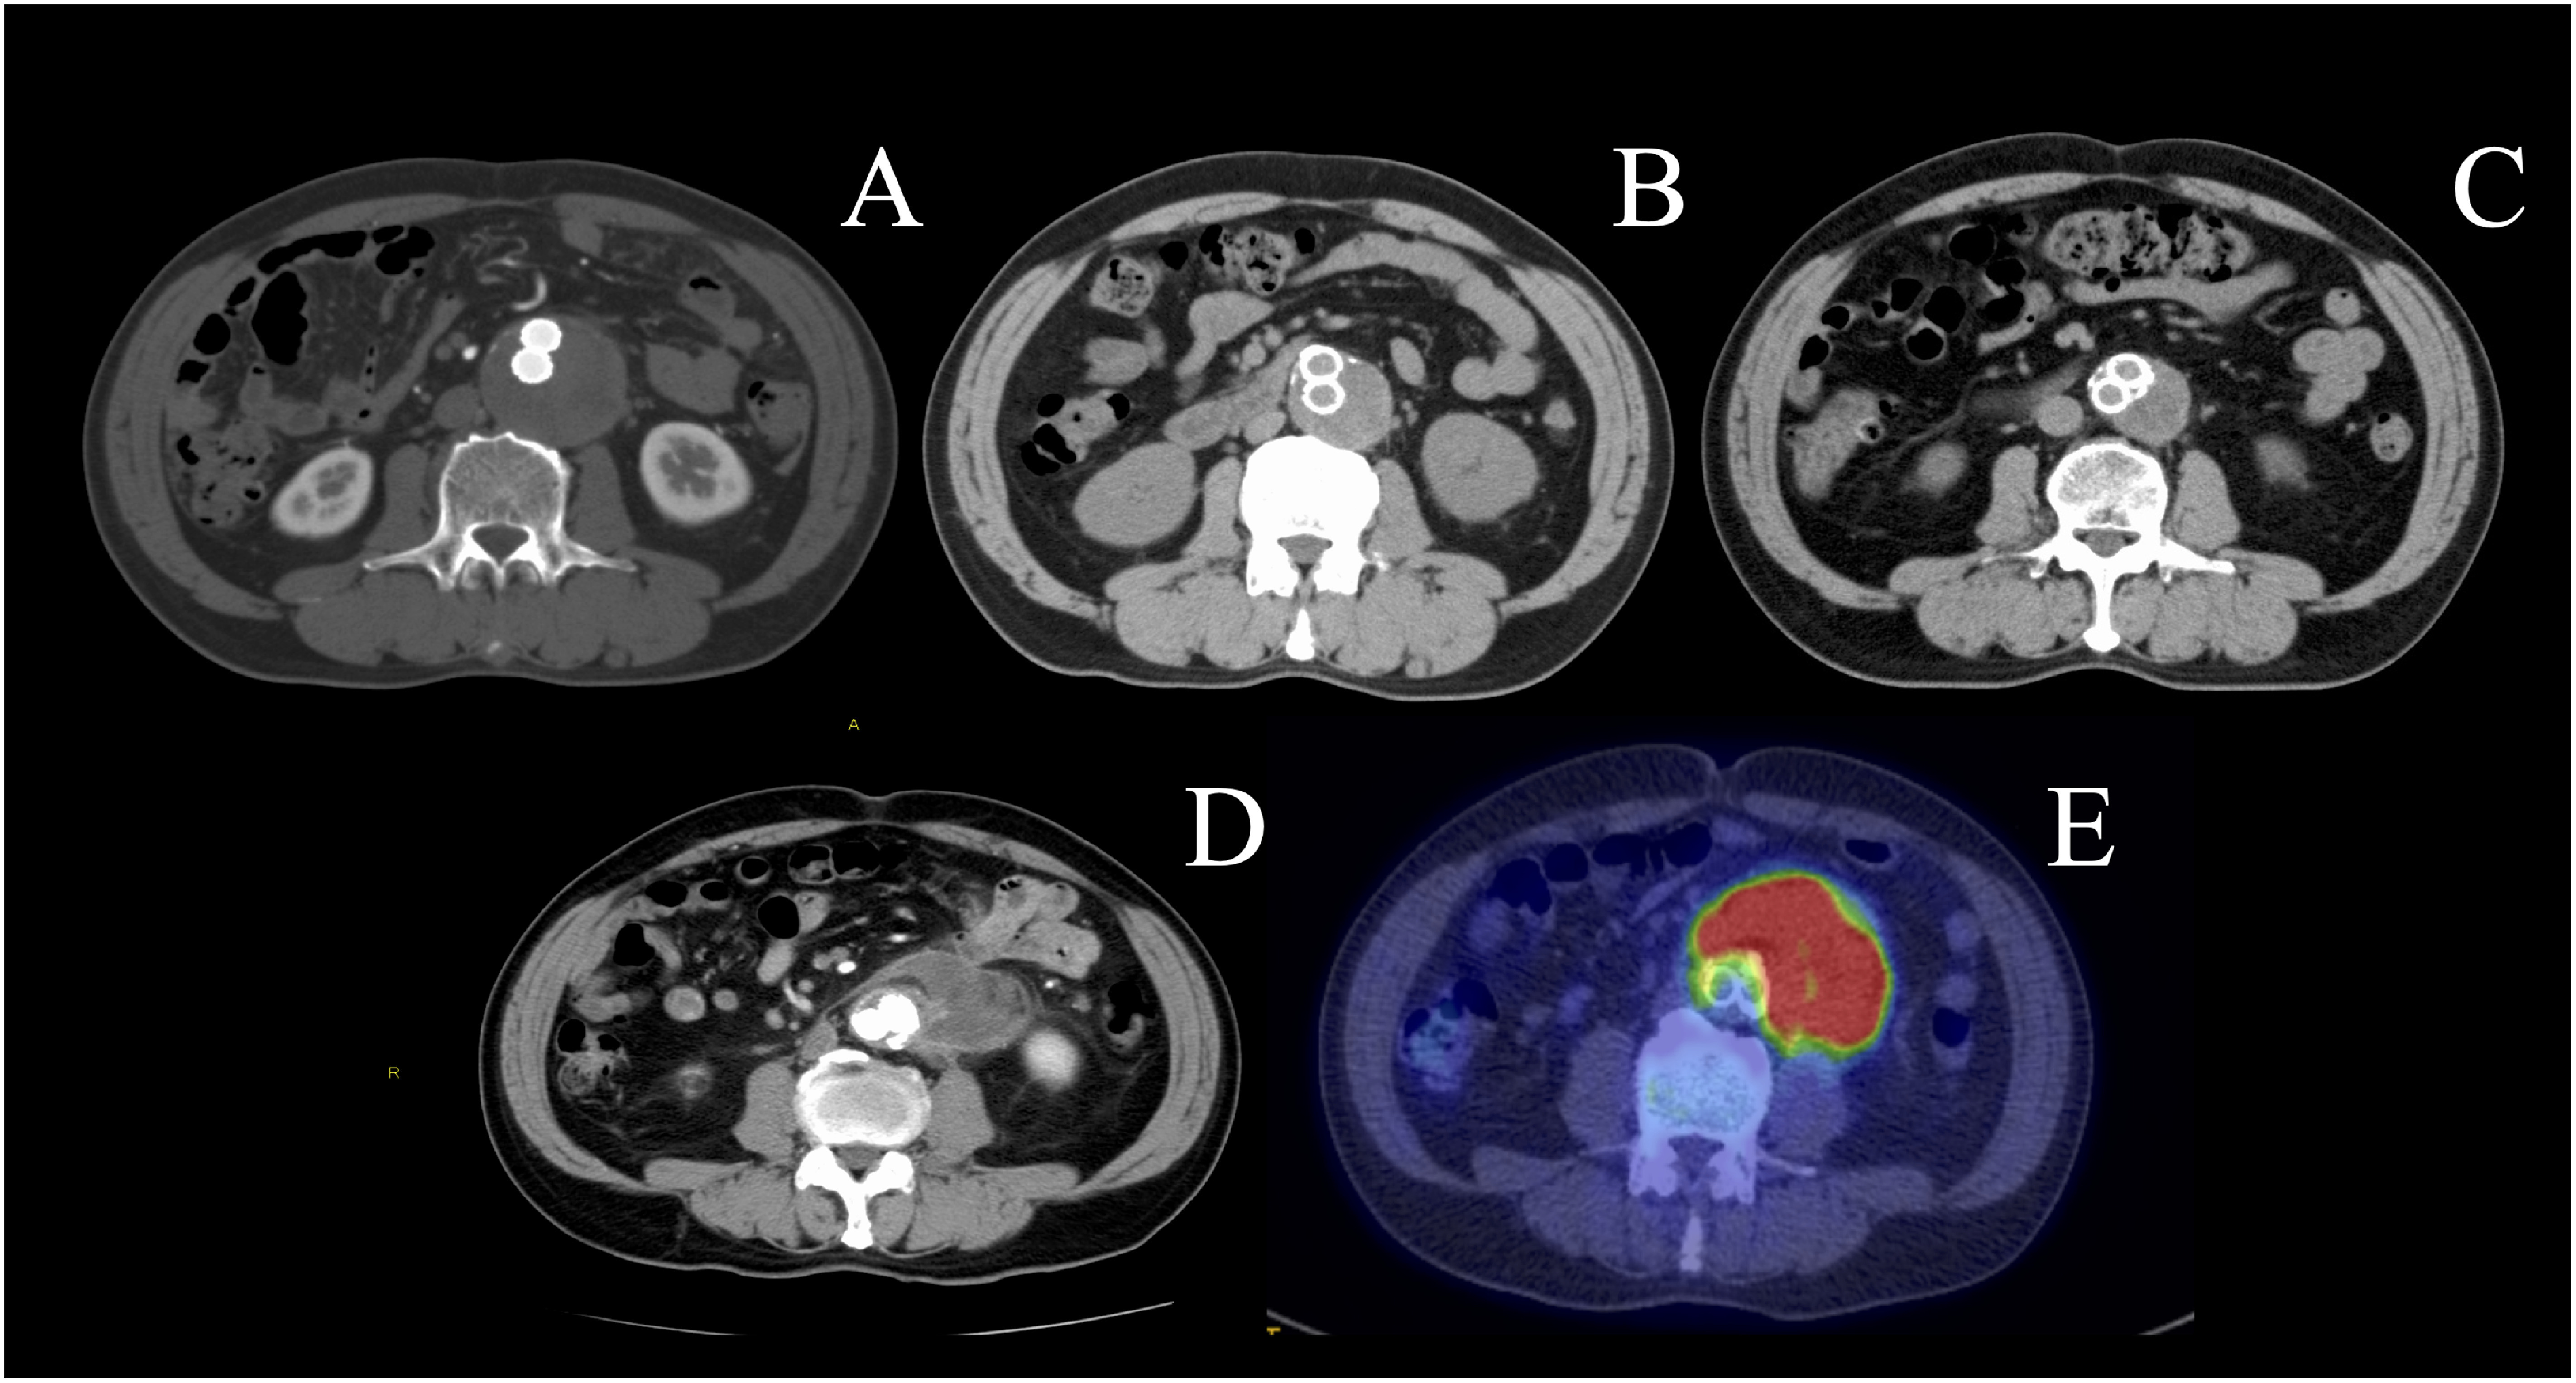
Fig. 2 Computed tomography (CT) showed the postoperative change of the aneurysm. (A) A half year after endovascular aortic repair, the diameter of the aneurysm was 47 mm. (B) The diameter of the aneurysm was 34 mm 2 years after endovascular aortic repair (EVAR). (C) The diameter of the aneurysm was 32 mm 3 years after EVAR. (D) At 3.5 years after EVAR, an enhanced CT showed a lobulated lesion around the stent-graft. (E) A positron emission tomography-CT showed a high ^18^F-fluorodeoxyglucose uptake of the aneurysm.
